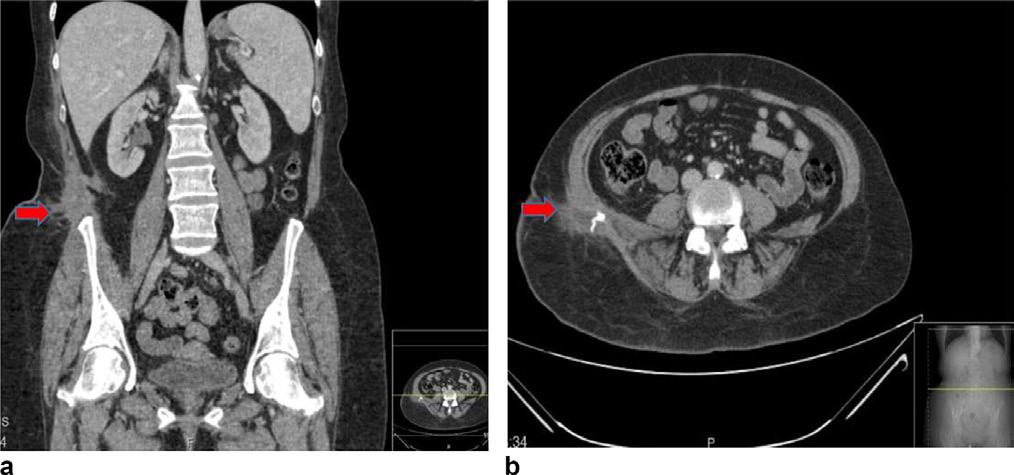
CT abdomen and pelvis with contrast 4 weeks after the procedure showing a small right residual collection in posterolateral abdominal wall/flank with no new collection or free fluid (red arrow).
